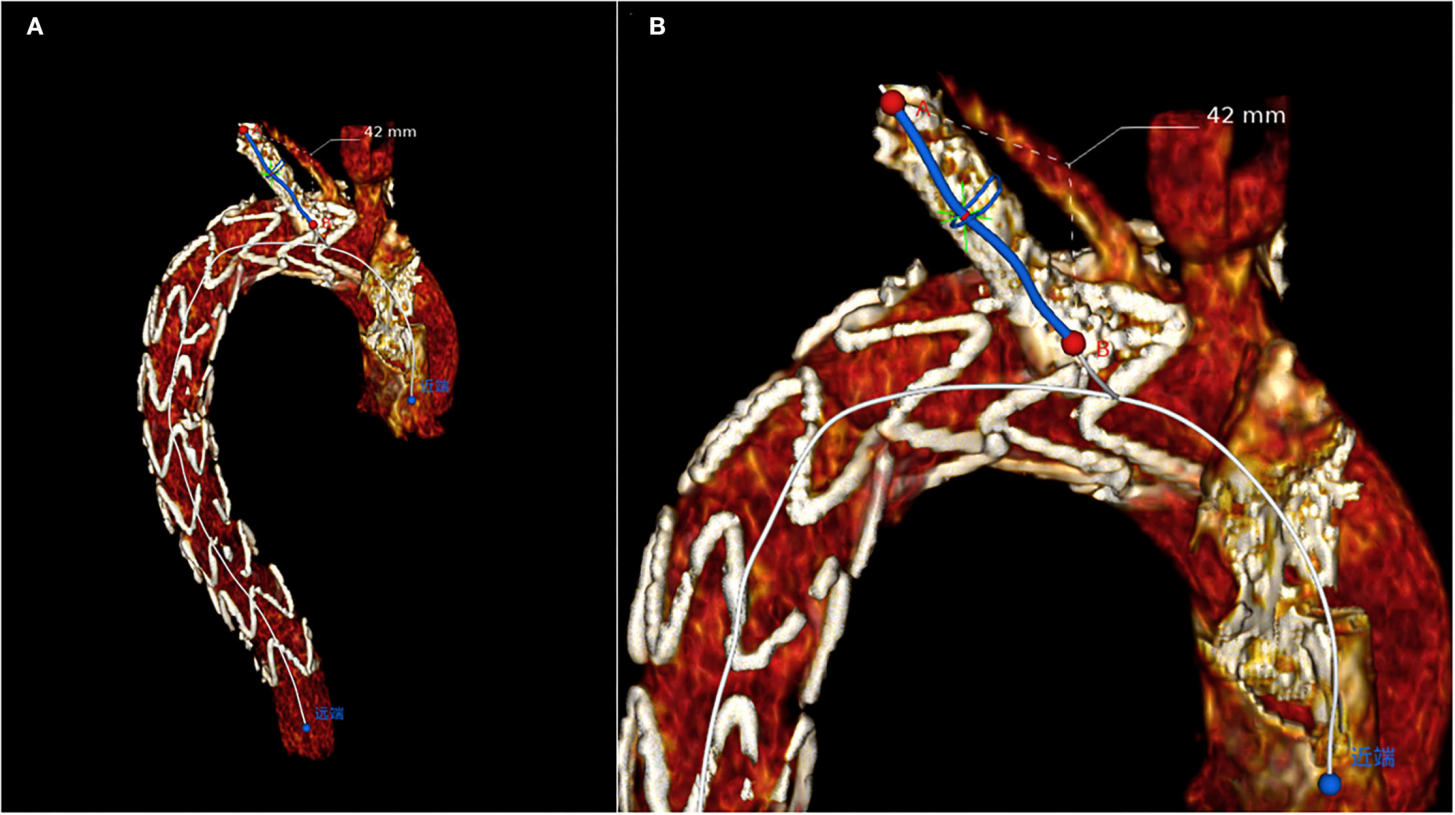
Plane of CTA image adjustment principle: Adjust the CTA image plane from all directions until the observer's line of sight is perpendicular to the LSA plane. (A) The overall view. (B) The magnified partial view.
